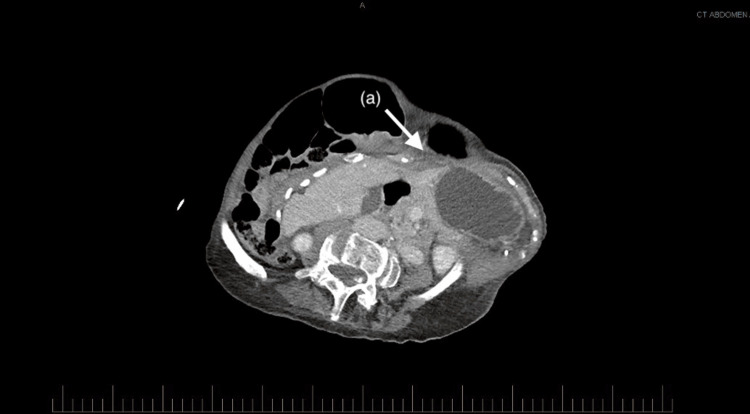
Axial slice of a CT abdomen and pelvis (a) Gastric outlet obstruction due to impingement by the right chest wall
